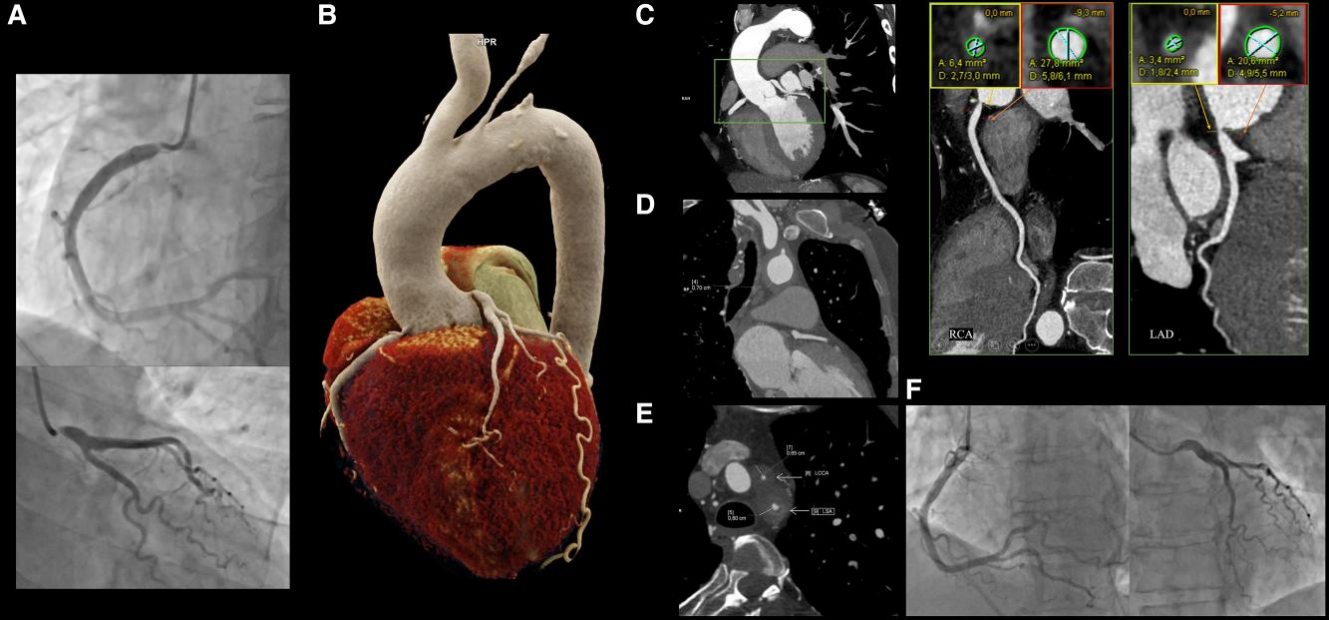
Imaging examinations. (*A*) ICA of right and left coronary arteries presenting ostial lesions. (*B*) Spatial volume rendering of CTA. Ostial lesions of coronary arteries are presented with total occlusion ofLSA and significant narrowing of LCCA. (*C*) Ostial lesions of coronary arteries presented in multiplanar reconstruction of CTA, including diameter measurements of stenosis and distal references for both coronaries. (*D*) Thickening of aortic wall up to 7 mm in CTA. (*E*) Narrowing of LSA and LCCA, with wall thickness 8 and 6 mm, respectively. (*F*) Final result of coronary angiography post-PCI.
